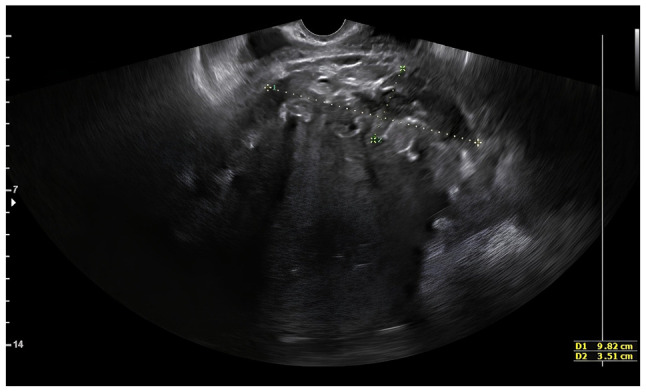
Transvaginal ultrasound image demonstrating a 9.8x3.5 cm mixed echogenic mass located in the pre-vesical space, at the level of the uterine scar, marked by two diameters.
